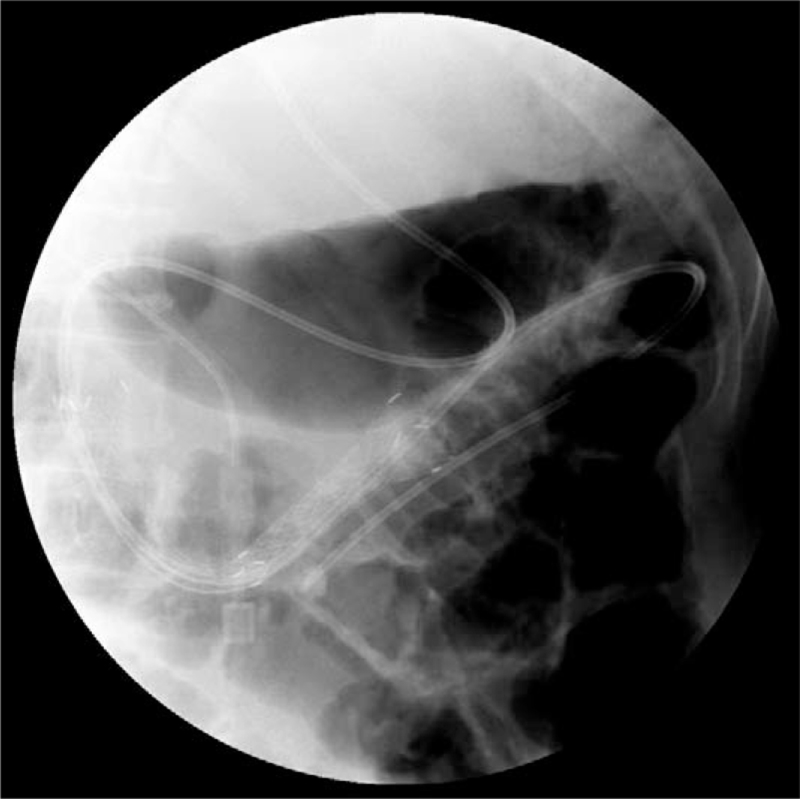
Abdominal radiograph showed that the 10-cm stent started in the 2nd part of the duodenum and ended in the 3rd part of the duodenum. The length of duodenal stenosis is nearly 7 cm and the diameter of the narrowest part was about 2 mm.
